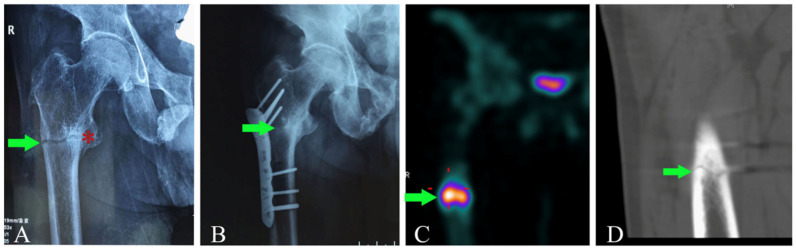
(A): Transverse fracture line of the proximal femur, with callus formation (star) as well as cortex breakage (arrow) simultaneously. (B): Successful healing of the primary pseudofracture of the proximal femur (arrow). (C): High uptake of 99mTc-MDP beneath the osteosynthesis plate, indicating a new pseudofracture of the proximal femur (arrow). (D): The transverse fracture line (arrow) revealed by computed tomography scanning (as reproduced with permission from ref. [27]).
